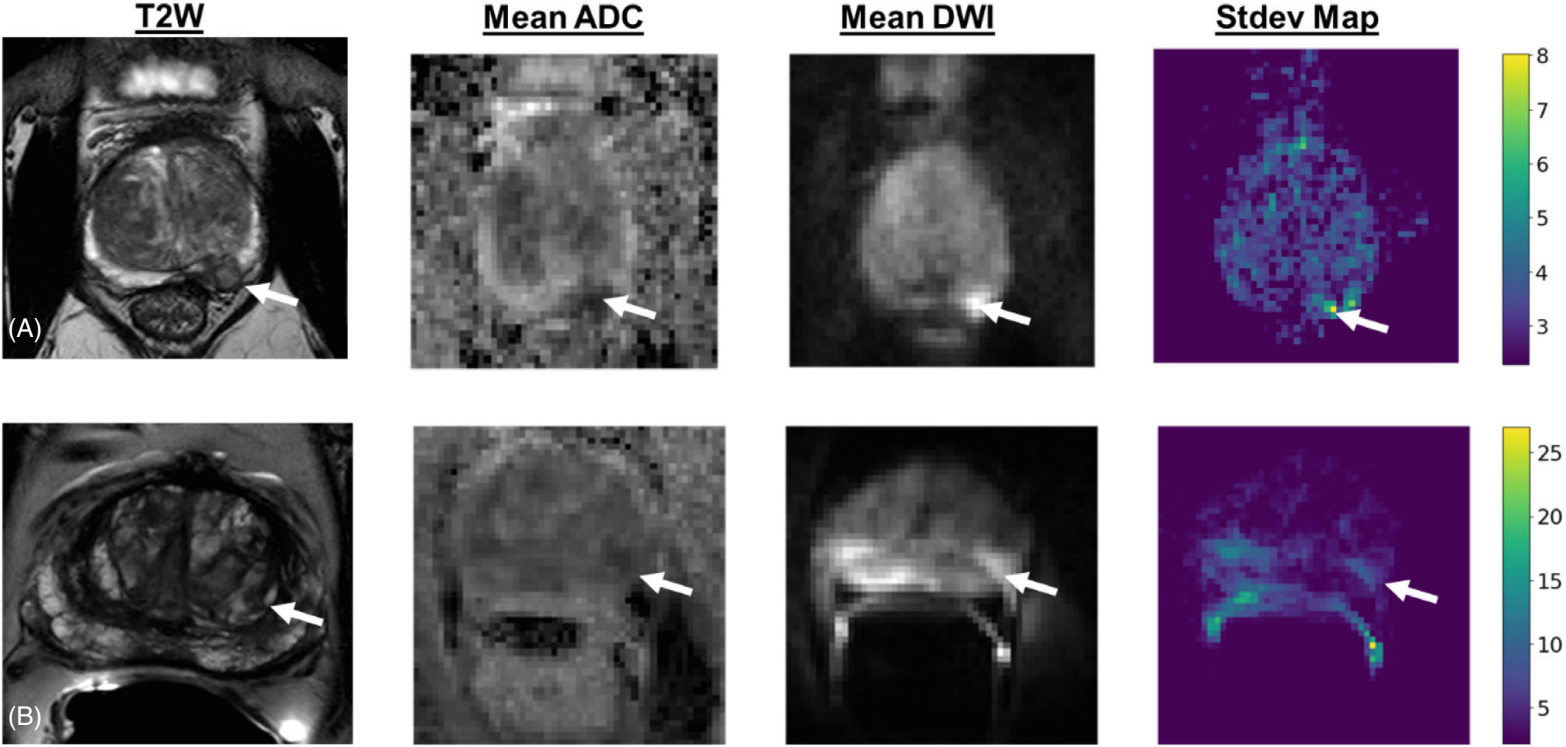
T2W, mean apparent diffusion coefficient map, mean diffusion‐weighted image (DWI) and corresponding DWI standard deviation for (A) a nonendorectal coil and (B) an endorectal coil patient
